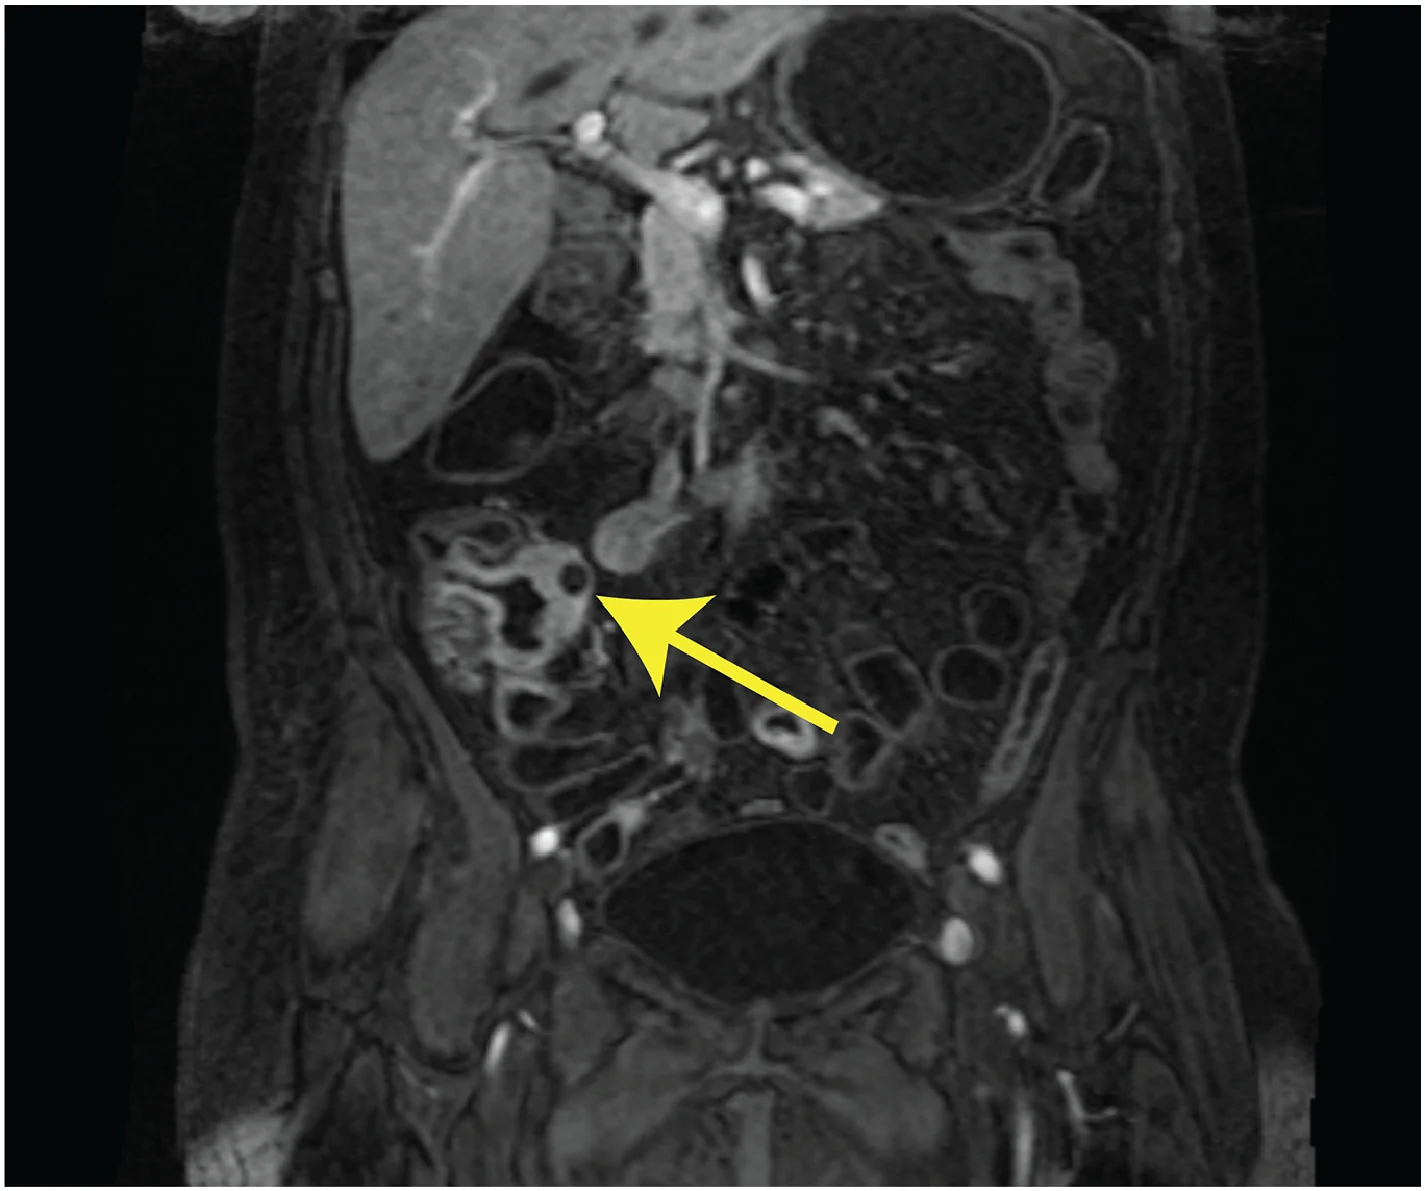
MR enterography of terminal ileum. Coronal T1-weighted contrast-enhanced MR enterography image demonstrates severe wall thickening of the terminal ileum with asymmetric mural enhancement and ulcerations (yellow arrow).
